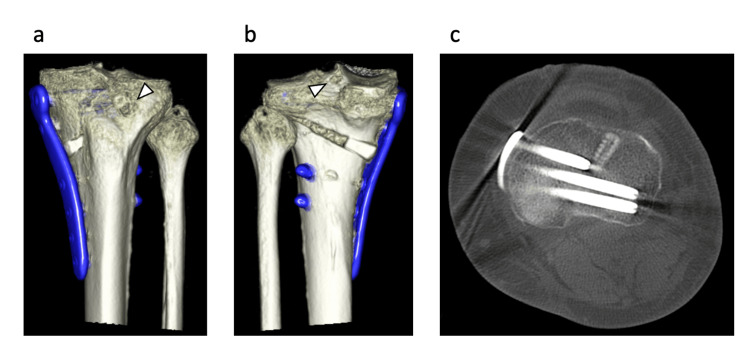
Our clinical case that underwent open-wedge high tibial osteotomy (OWHTO) and pull-out repair using the anatomical tibial tunnel (AT) (a) Three-dimensional CT image showing the distal hole of the AT, indicated by the arrowhead. (b) Three-dimensional CT image showing the proximal hole of the AT (at the medial meniscus posterior root attachment site), indicated by the arrowhead. (c) Axial CT image demonstrating that the anatomical tibial tunnel intersects with the anterior screw.
